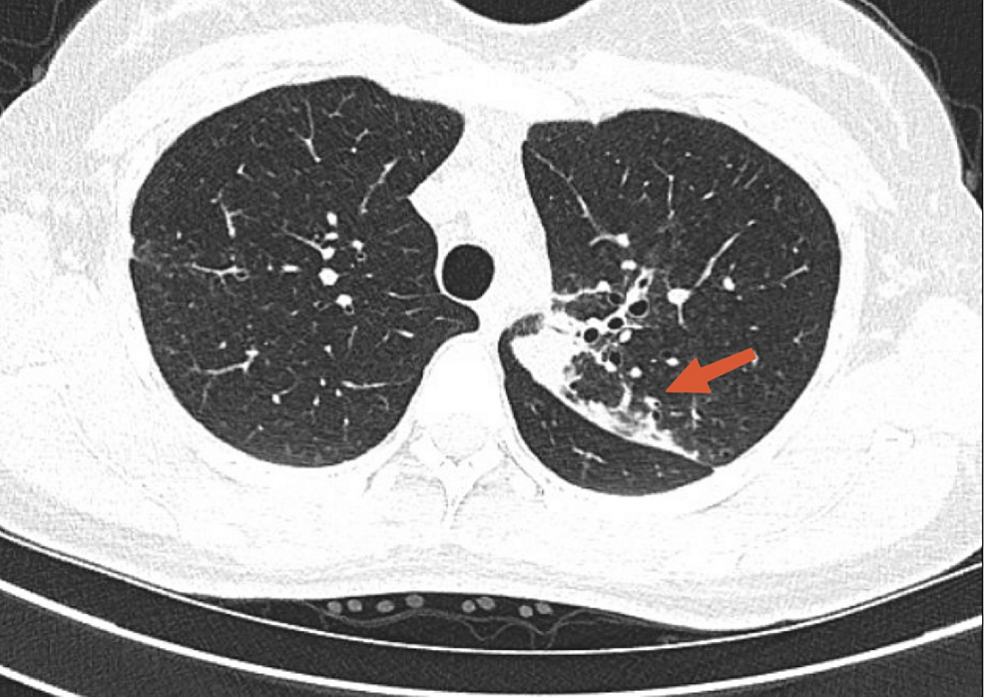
CT of the chest without contrast demonstrating linear areas of scarring involving both lung apices and more prominent on the left side (red arrow).
